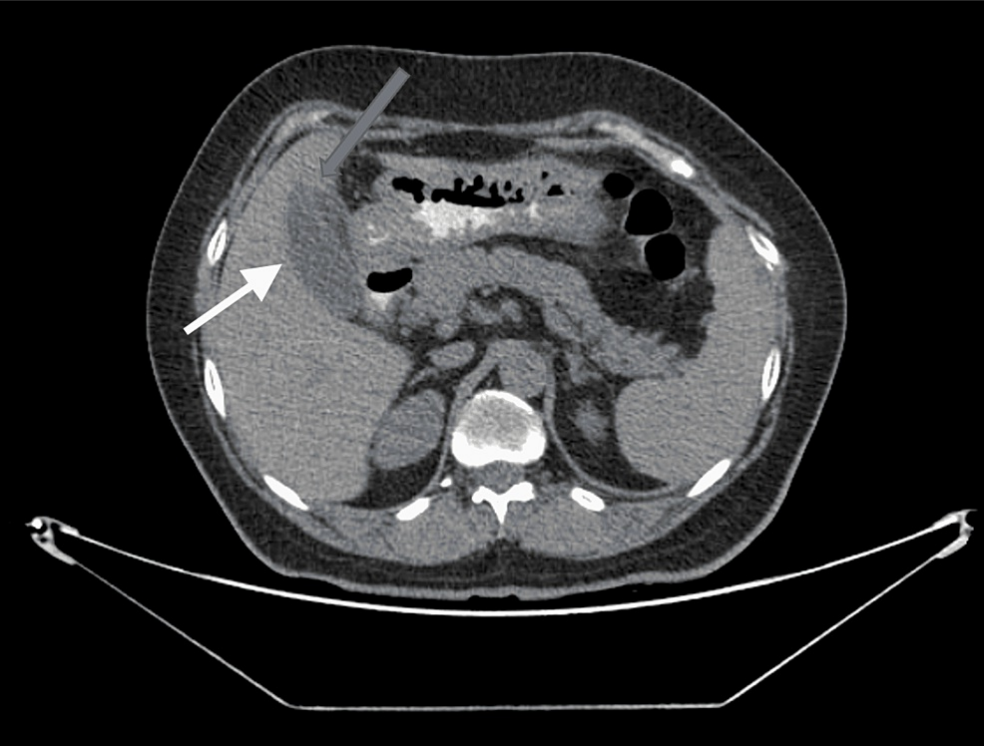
CT abdomen axial view showing the duplicated gall bladder, one of them with the collapsed lumen (grey arrow) and distended gall bladder (white arrow)
